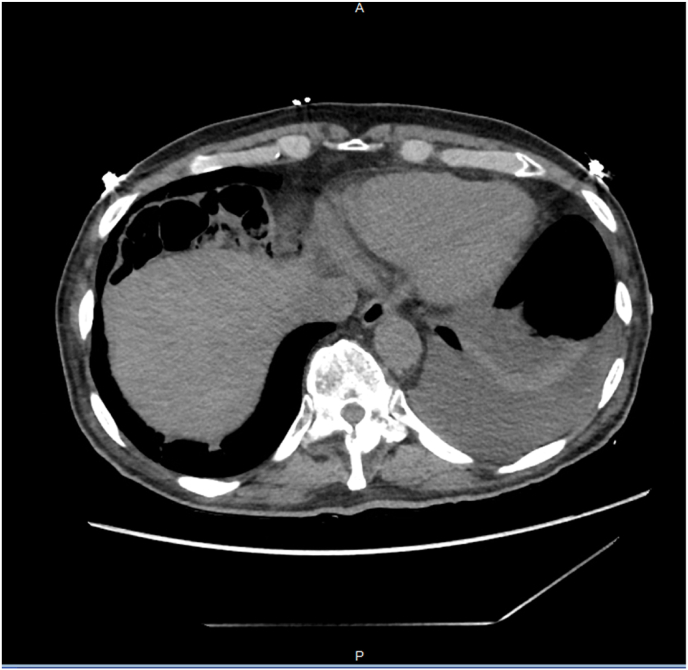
Computed tomography of chest without contrast revealing Chilaiditi's sign and absence of pulmonary embolism.
